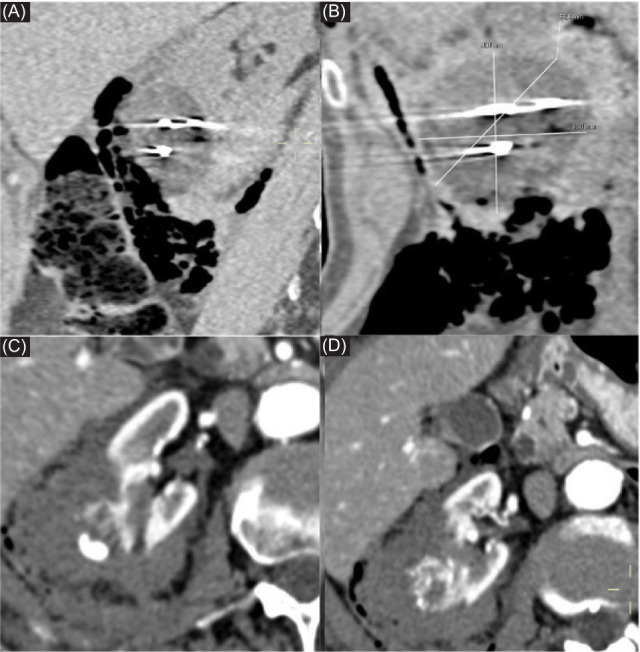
CT scans in (A) axial and (B) coronal reconstruction during the procedure established the intralesional positioning of five cryoprobes and the dissection with air obtained to separate the bowel from the ablation zone. (C, D) CT scans at the end of the procedure depicted perirenal hematoma without active bleeding.
